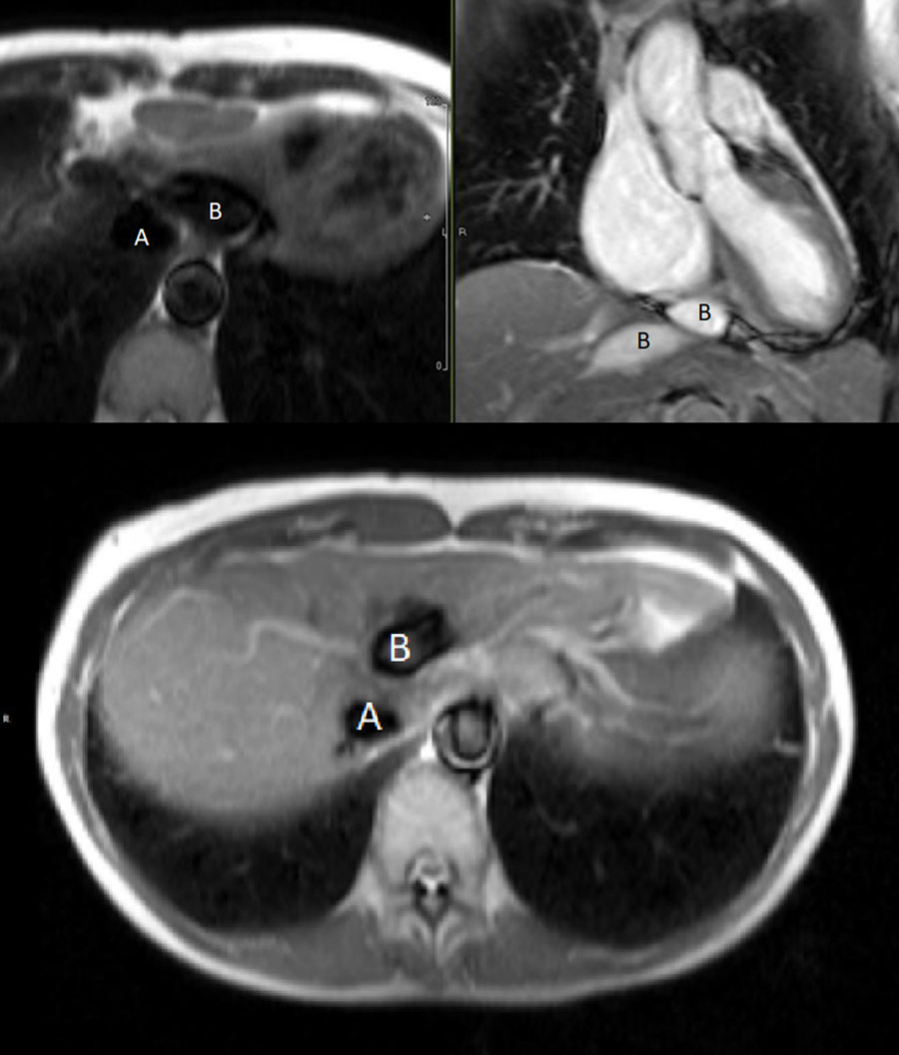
Magnetic resonance images of incidental Abernethy malformation. Axial half Fourier single‐shot turbo spin‐echo (top‐left and bottom) and coronal fast imaging with steady‐state precession (top right) images demonstrating the inferior vena cava (A) and extrahepatic portosystemic shunt (B)
